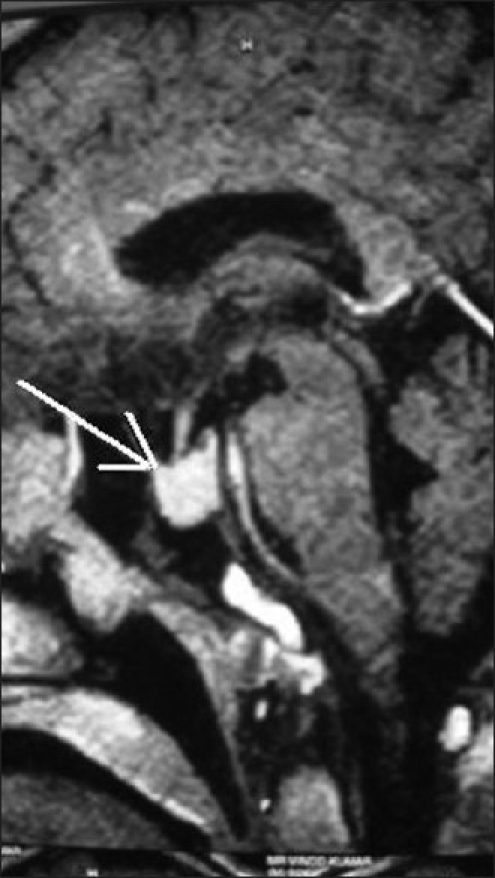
Contrast enhanced MRI scan (saggital section) - intrasellar pituitary macroadenoma (white arrow) involving the anterior lobe of the pituitary with no parasellar or suprasellar extension
